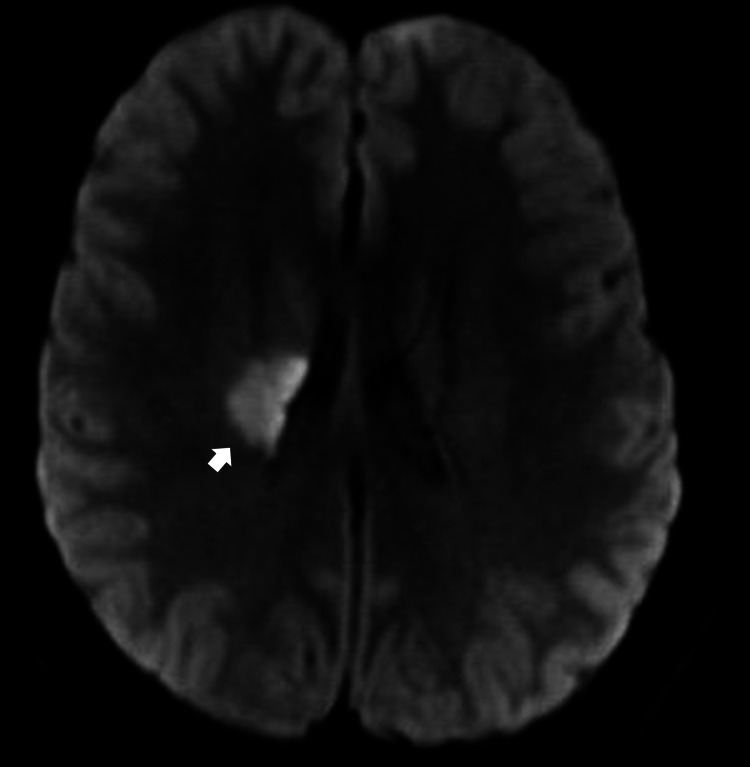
Axial slice in T FLAIR sequence shows a hyperintense area located in the tail of the right putamen and the posterior limb of the ipsilateral internal capsule and extending toward the corona radiata. FLAIR: fluid-attenuated inversion recovery
